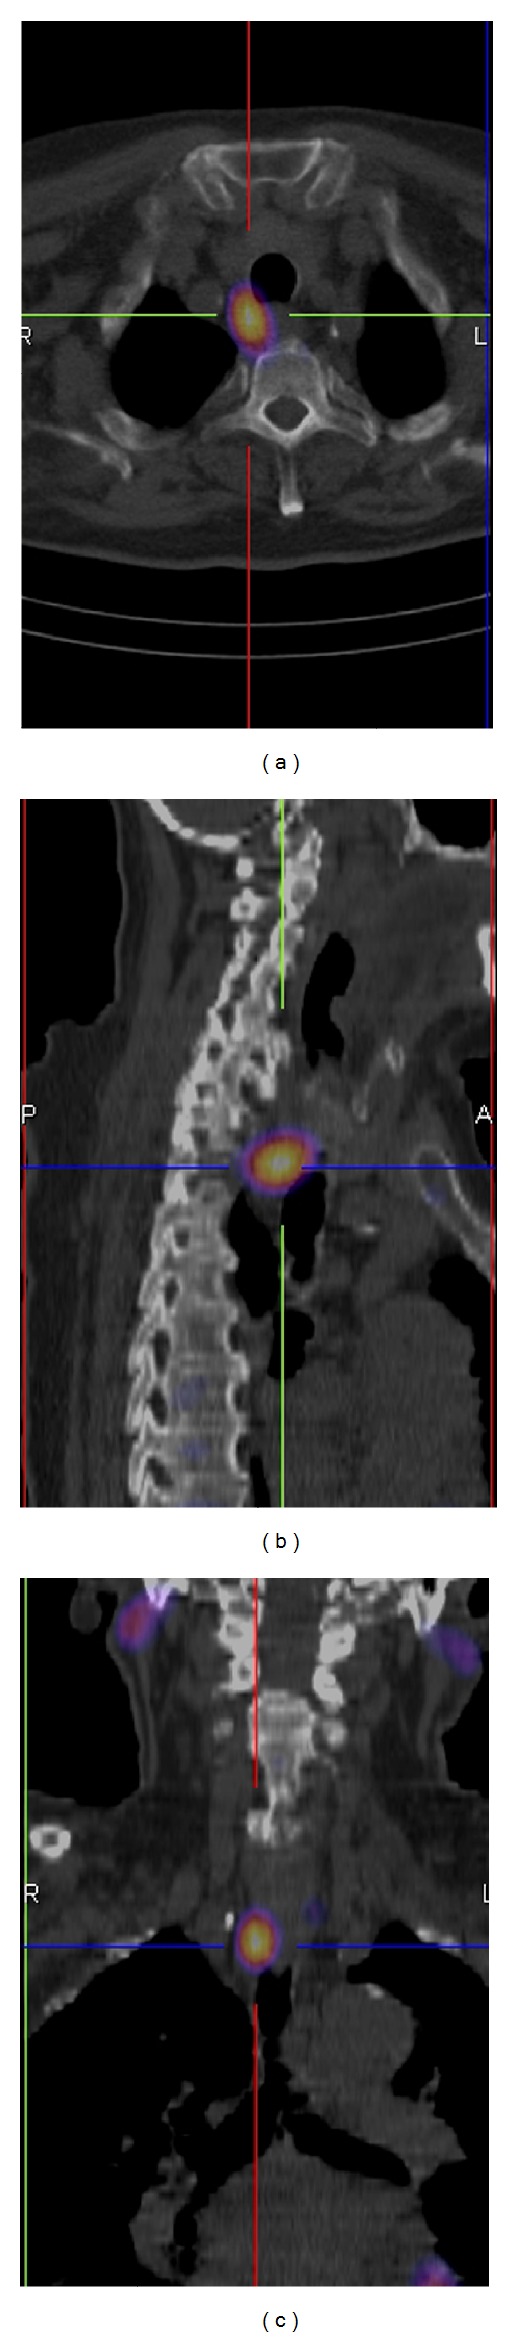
A ^99m^Tc-sestamibi uptake in a parathyroid adenoma located behind the trachea. ^123^I/^99m^Tc-sestamibi subtraction SPECT/CT images (transversal (a), sagittal (b), and coronal (c)).
